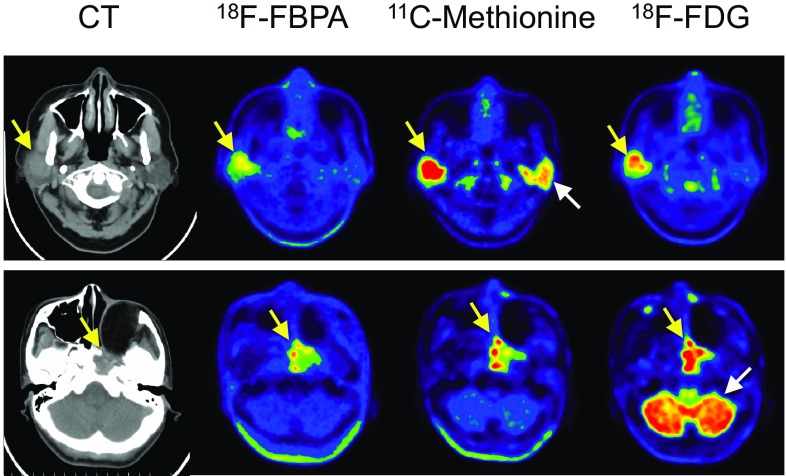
^18^F-FBPA, ^11^C-methionine, and ^18^F-FDG PET/CT images of patients with salivary gland duct carcinoma (upper row) and squamous cell carcinoma (lower row). Three PET scans were performed during a 2-week interval in the Southern TOHOKU Research Institute for Neuroscience (unpublished data, approved by the institutional ethics committee #224). The PET/CT scanner used was Discovery PET/CT 610 (GE Healthcare, Milwaukee, WI), and the injected radioactivity doses of ^18^F-FBPA, ^11^C-methionine, and ^18^F-FDG (upper low and lower row) were 4.3 and 4.6, 6.7 and 7.4, and 3.6 and 3.6 MBq/kg body weight, respectively. Yellow arrows; carcinomas; white arrows, salivary gland (upper row) and cerebellum (lower row). PET images are shown in the scale of SUV 0–6 except for ^18^F-FDG in the lower row (SUV 0–10). SUV_max_ values of ^18^F-FBPA (40–60 min post injection), ^11^C-methionine (20–30 min post injection), and ^18^F-FDG (50–60 min post injection) were 3.6, 9.7, and 7.1, respectively, in the upper row, and 5.9, 7.2, and 18.5, respectively, in the lower row
